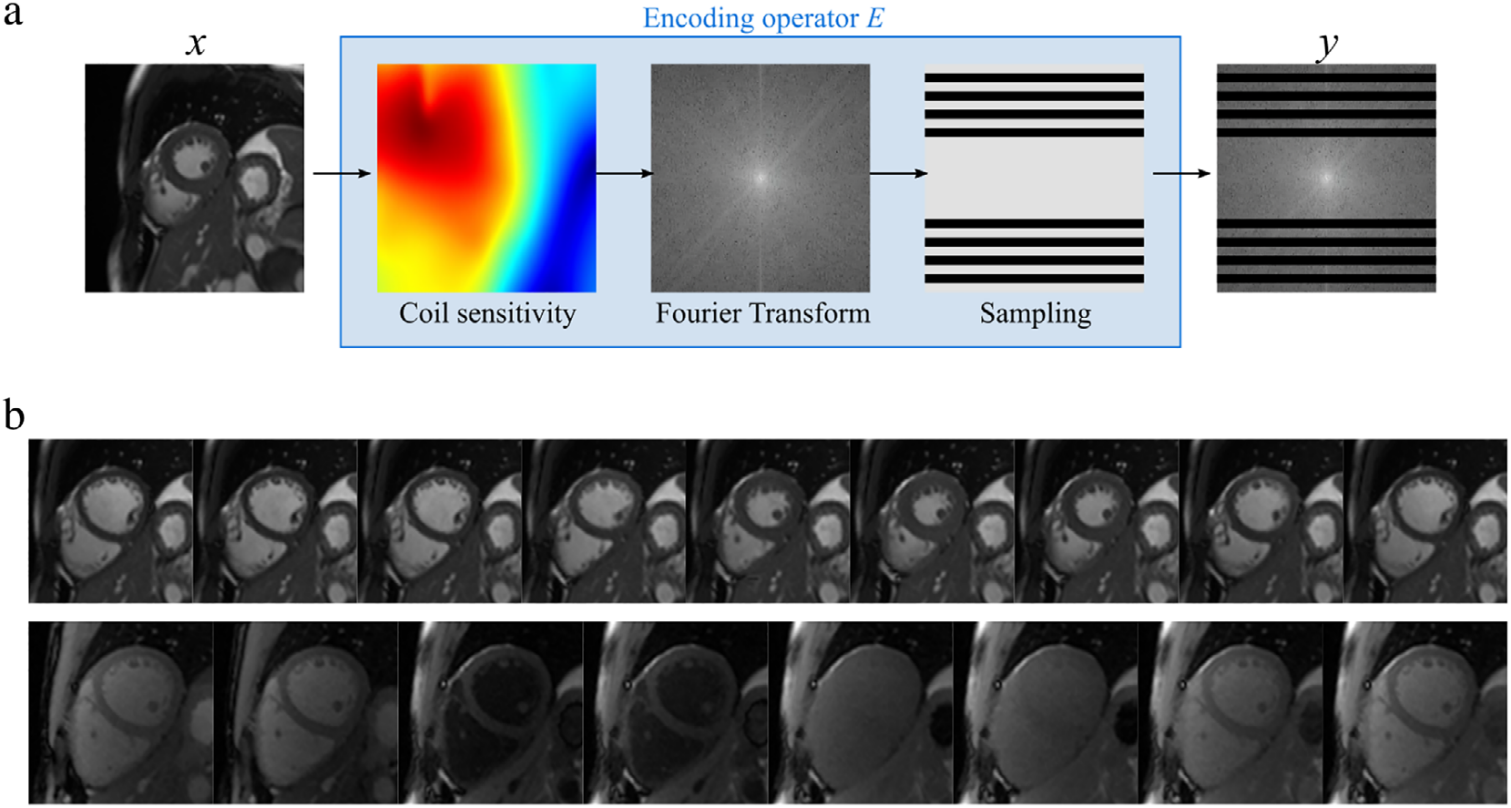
(a) MR measurement (i.e. forward) model, where x is the object being scanned, E is the MR encoding operator, including coil sensitivity profiles, Fourier transform and *k*‐space sampling mask, and y is the measured k‐space data. (b) Example MR images where x represents a multidimensional object, for example, a series of cardiac cine images (top), or a set of T_1_‐weighted images for cardiac T_1_ mapping (bottom).
